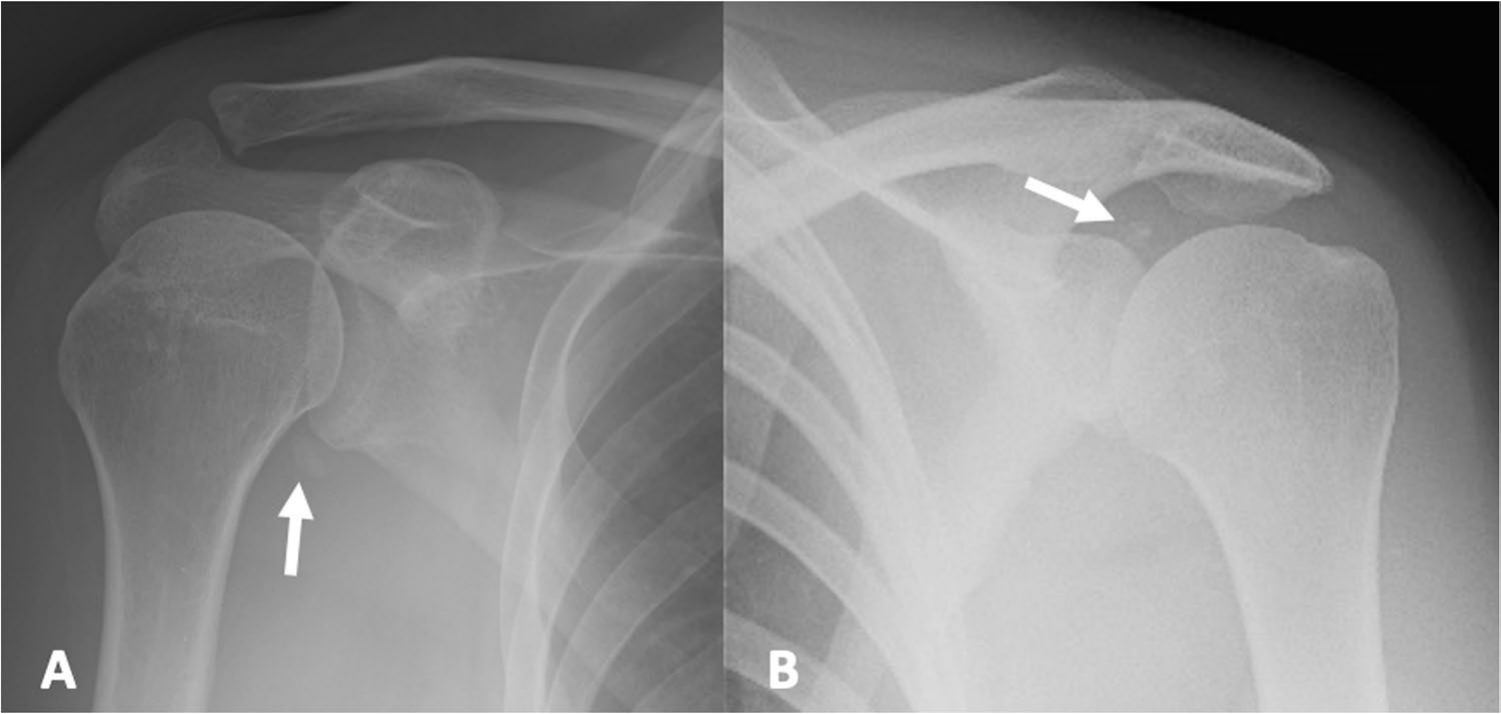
A 56-year-old female presented with a 10-day history of right shoulder pain radiating to the elbow. A AP radiograph of the right shoulder shows a focus of calcification in the region of the axillary recess/long head of triceps (arrow). B 41-year old female presented with a 3 day history of severe shoulder pain, with onset after a “pulling” injury to the shoulder. AP radiograph of the left shoulder demonstrates a rounded focus of calcification adjacent to the posterosuperior glenoid (arrow). MRI performed on the same day confirmed the diagnosis of ACP
